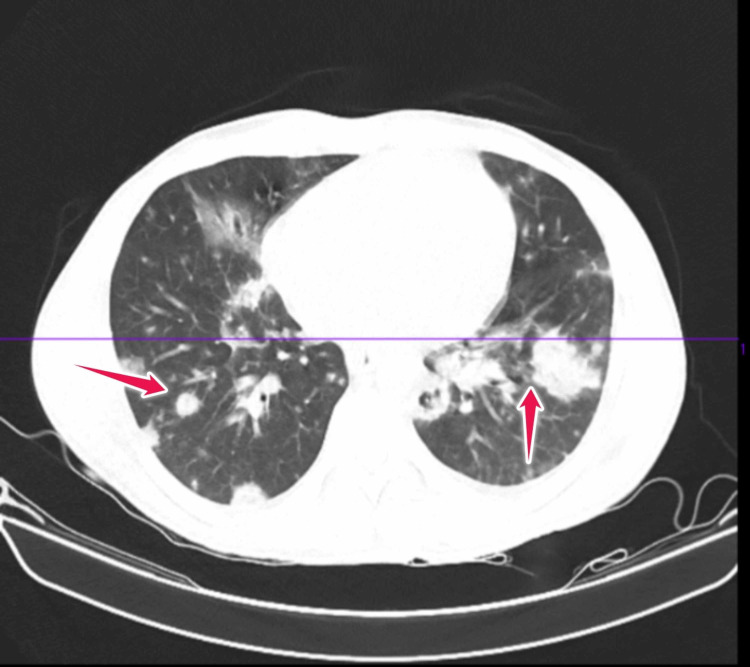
CT scan of chest done at the time of admission CT: computed tomography
